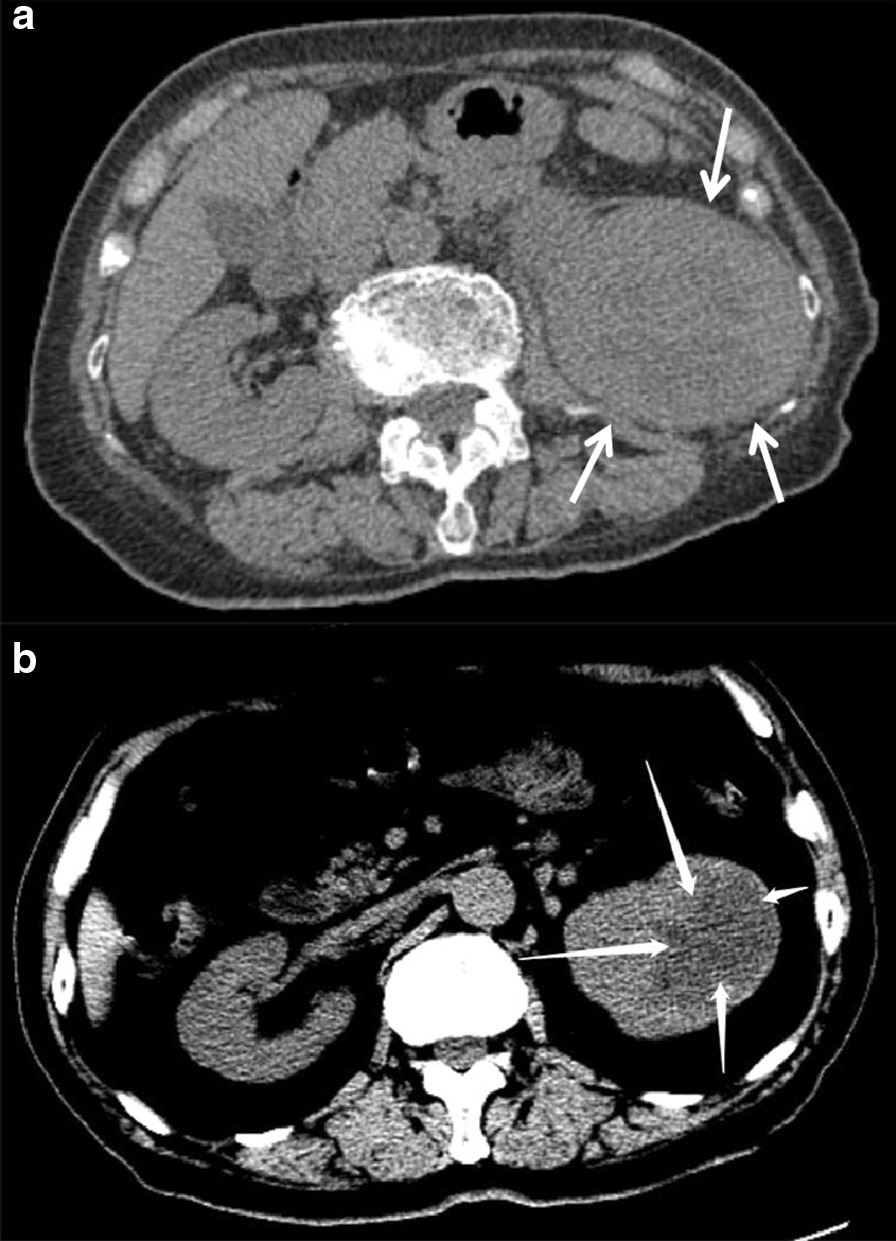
a Case 3: axial unenhanced CT image shows a significant increase in the volume of the left kidney CDC. The left medulla is unclear. The lesions are mainly solid components (arrows). b Case 6: axial unenhanced CT image shows mixed density in the left kidney and a clear cystic low-density lesion with a clear border (arrows)
